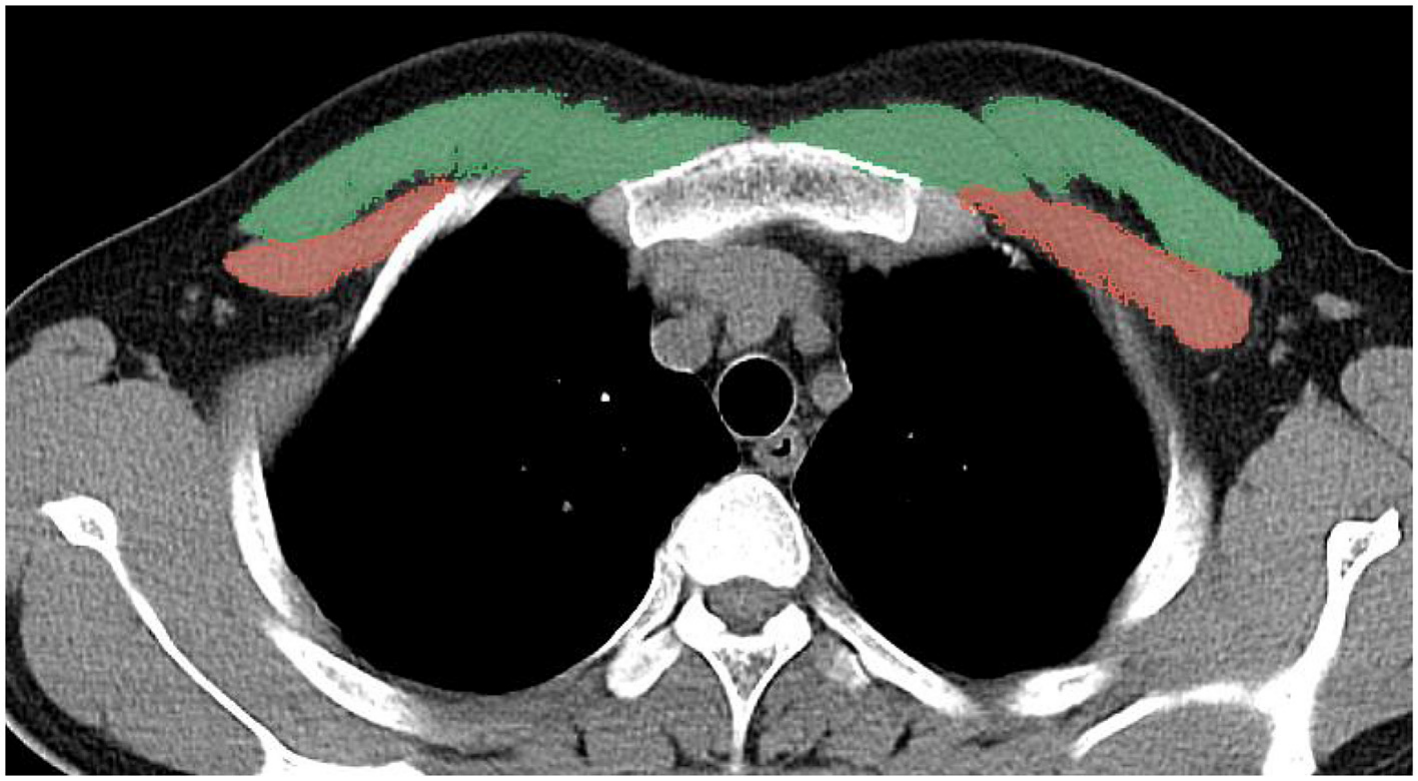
A method for determining PMA by CT horizontal plane of the T4 vertebrae, the pectoralis major is green and the pectoralis minor is red.
